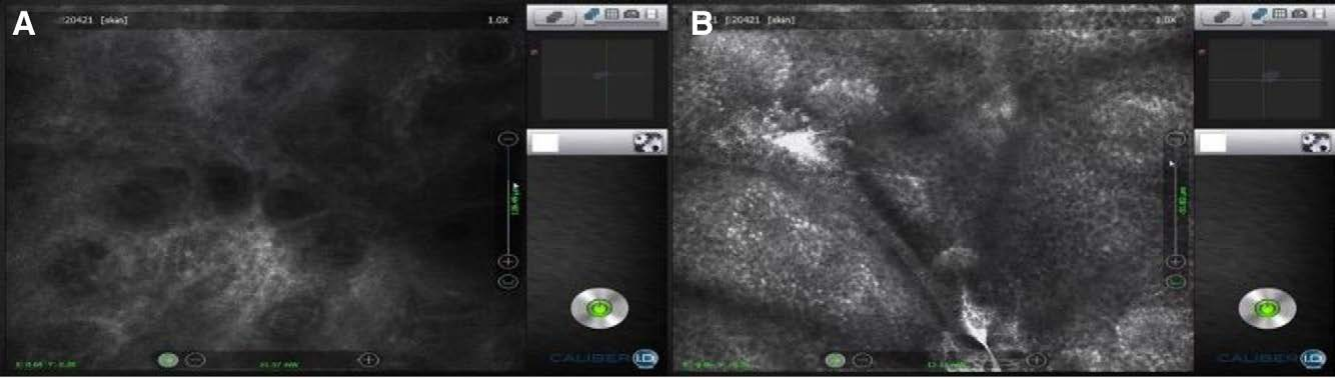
Skin CT (A, B) showed that compared with the surrounding normal skin, the white spine area was atrophied, the pigment ring in the basal layer was missing, and inflammatory cell infiltration was seen in the dermal papilla. CT = computed tomography.
